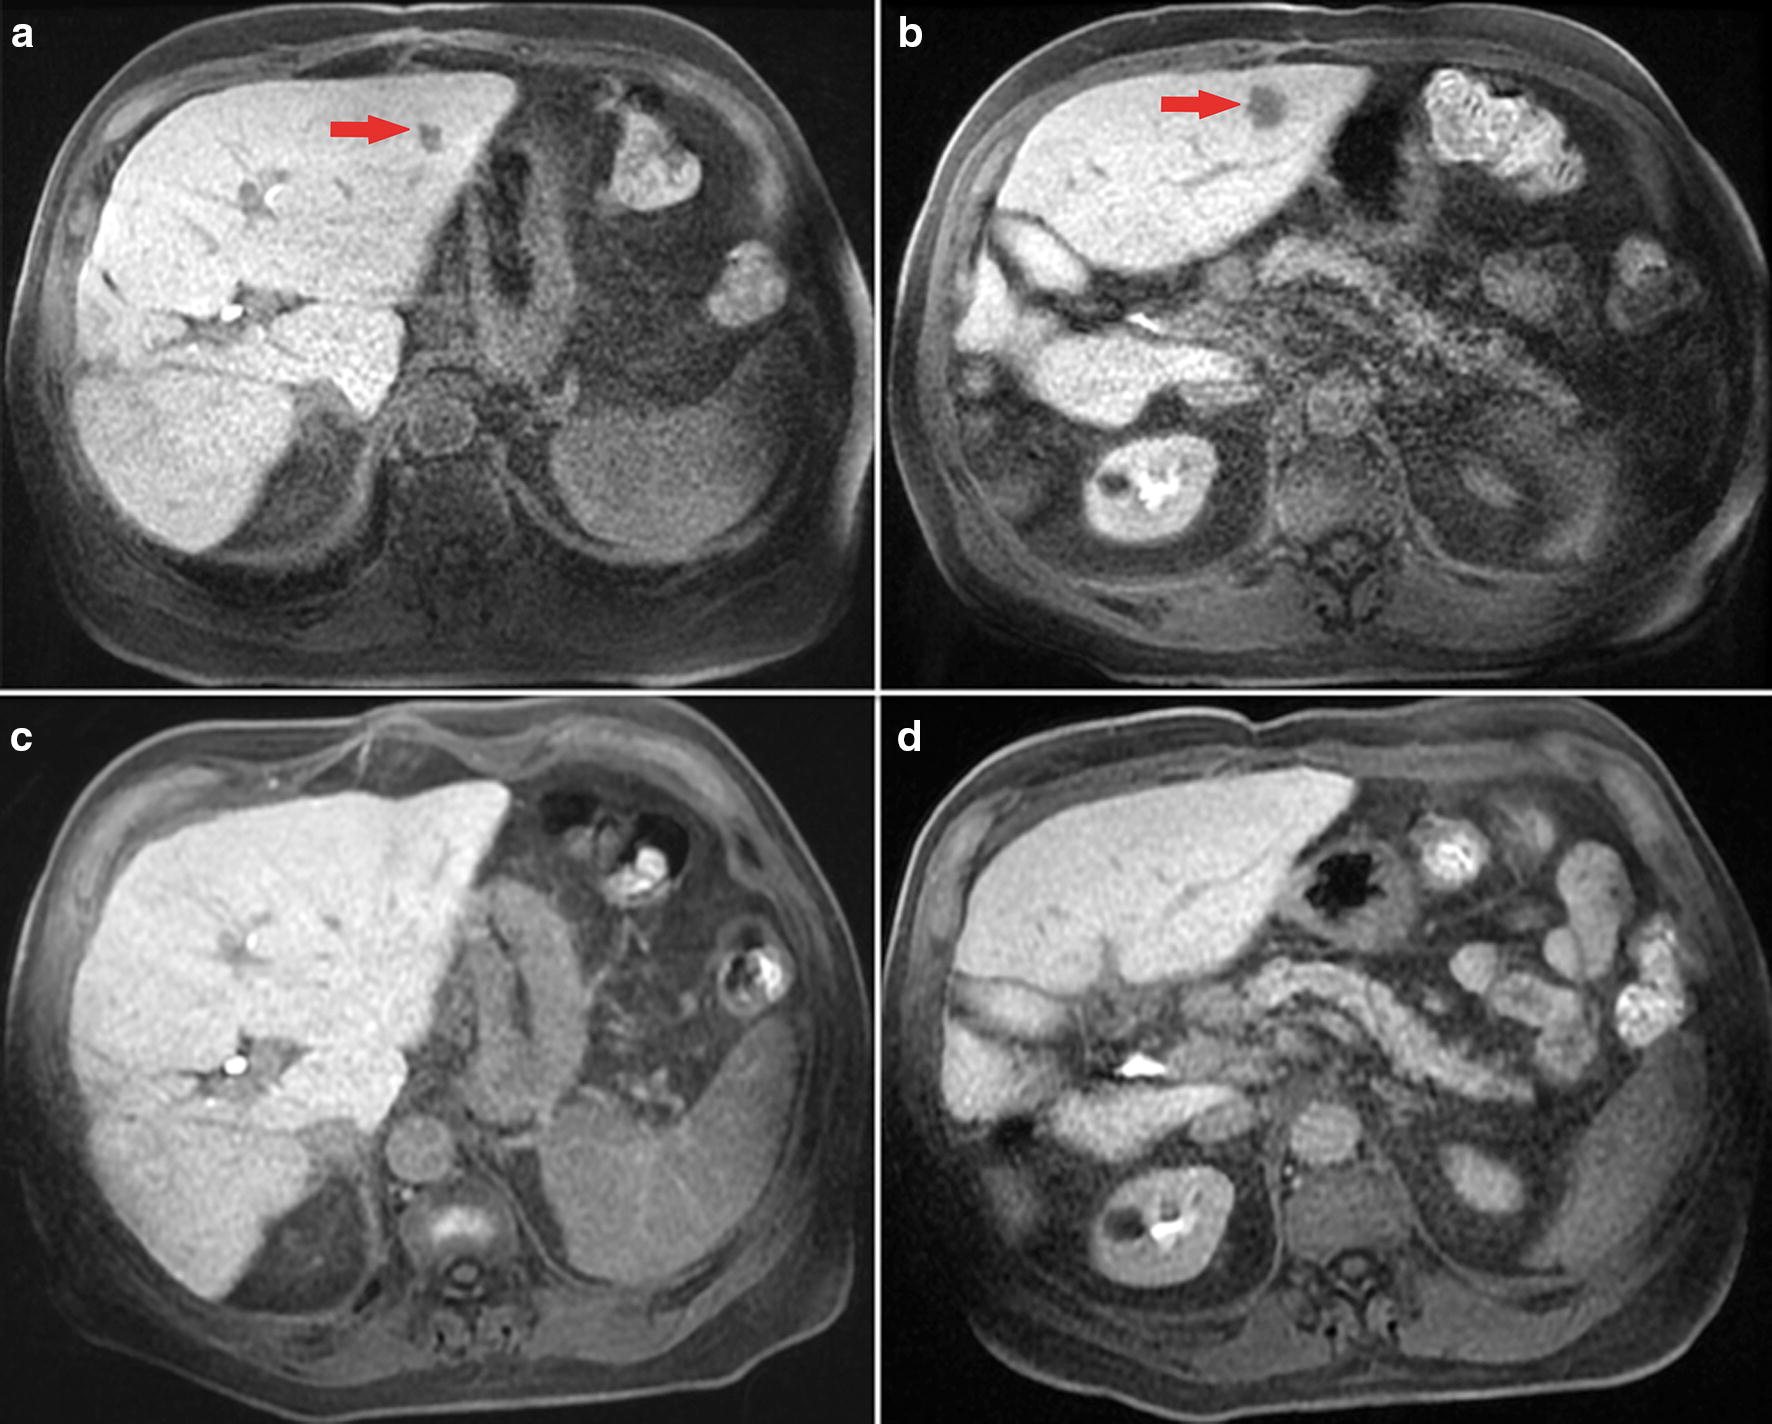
Lesions in case 1. Gd-EOB-DTPA-enhanced MR images showing, at baseline acquisition, hepatobiliary phase 4 millimetric lesions: a 10 mm hepatocellular carcinoma (HCC) nodule in segment II (a), a 14 mm HCC nodule in segment III (b), and a millimetric lesion at segments V and I. Gd-EOB-DTPA-enhanced MR follow-up after 89 months since starting systemic treatment with metronomic capecitabine shows a stable complete response, with the absence of both HCC nodules (c, d)
